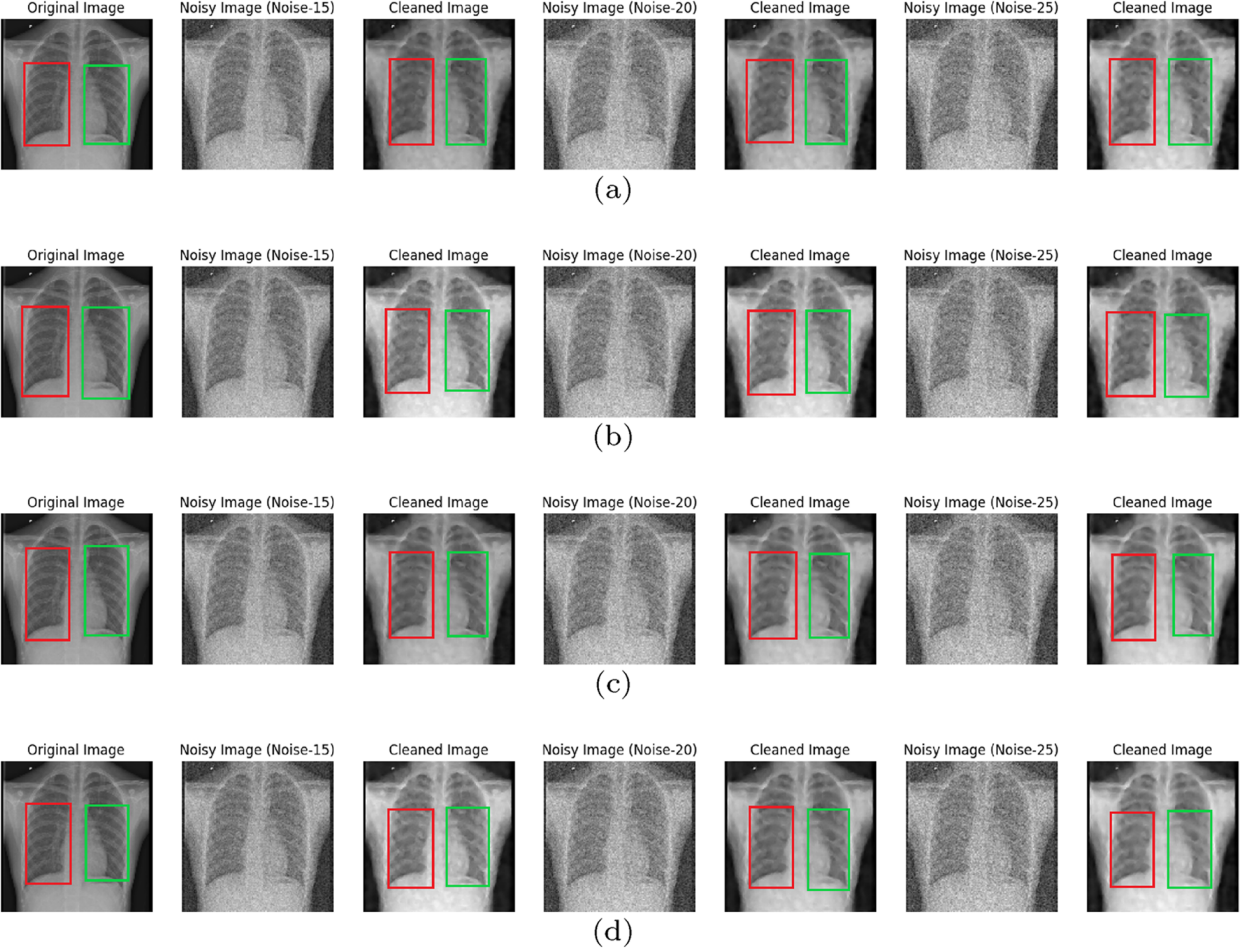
Original X-Ray image with noisy($$N_{sp}$$=15,20,25 and $$N_{bl}$$=0 to 55) and denoised or cleaned image by DMF-Net where (a and c) shows for specific noise without and with augmentation in (b and d) shows for blind noise without and with augmentation; red box and green box to observe left lungs and right lungs area respectively with original image
